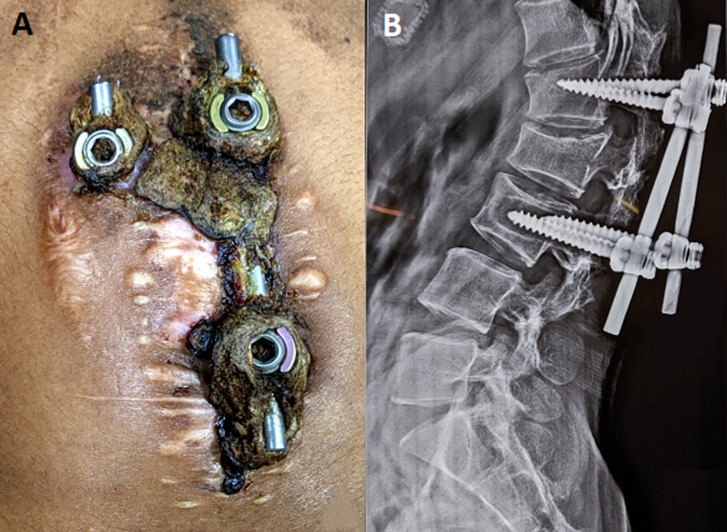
A) exposed implants protruding from lumbar region, surrounded by dry necrotic tissue; B) X-ray lateral view of the lumbar spine suggestive of retracted implants
